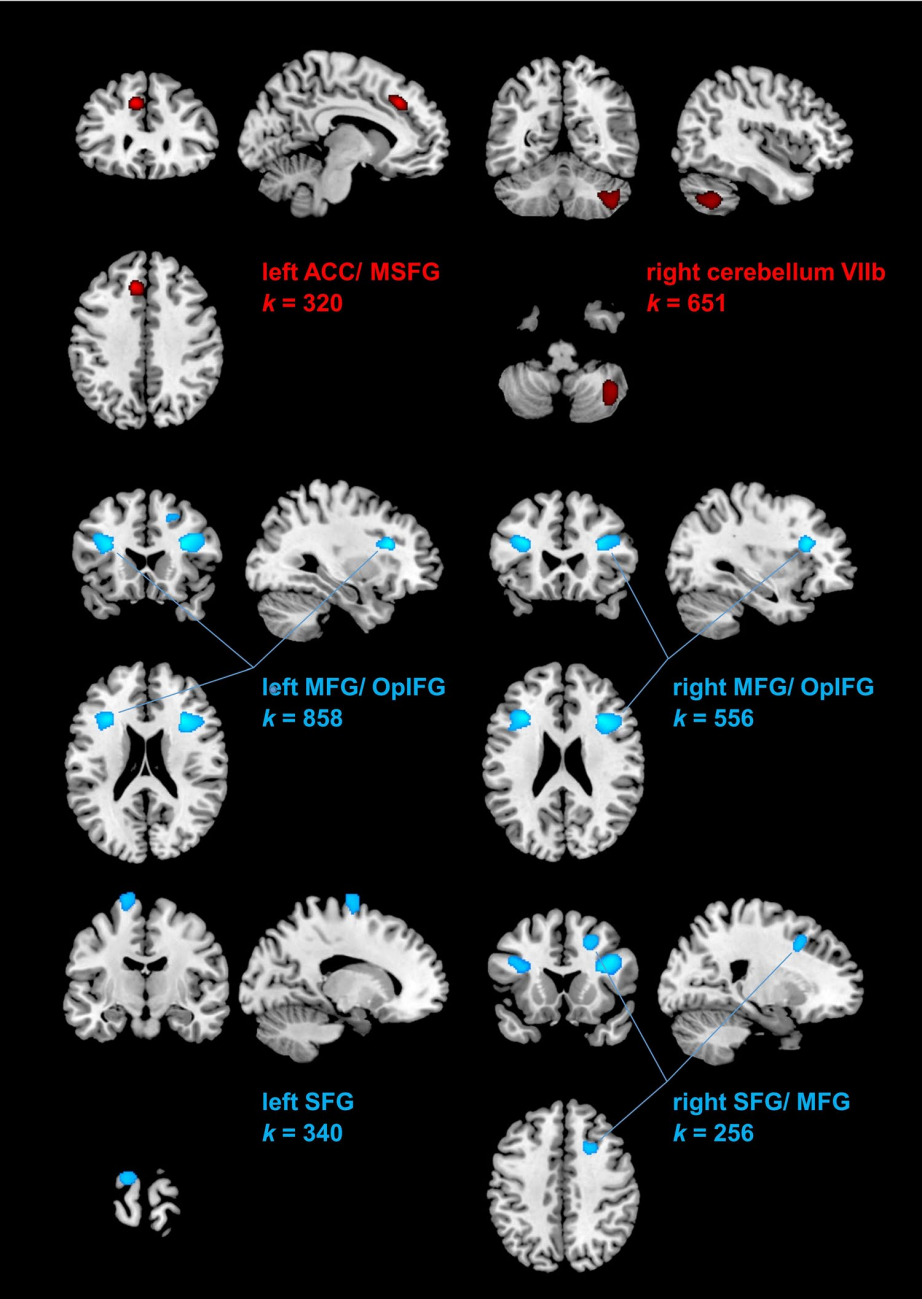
Graphical depiction of identified significant clusters in the VBM analysis of increases in rGMV (in red): in the left anterior cingulate cortex (ACC)/medial segment of superior frontal gyrus (MSFG) and in the right cerebellum VIIb/Crus II/Crus II, as well as decreases in rGMV (in blue): in the left and right middle frontal gyrus (MFG) adjacent to the opercular part of the inferior frontal gyrus (OpIFG), and in the left and right superior frontal gyrus (SFG), in ultrasound verum, relative to placebo.
